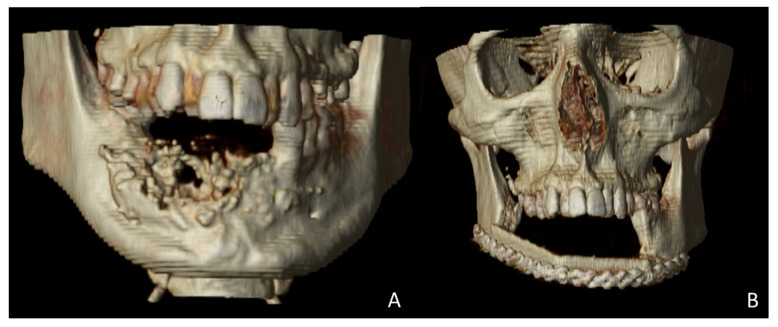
Postoperative 3D scan. (A) CT Scan showing destruction of the mandibular symphysis due to ameloblastoma. (B) CT Scan with segmental mandibulectomy from left mandibular body to right mandibular angle and immediate reconstruction with fibula free flap.
